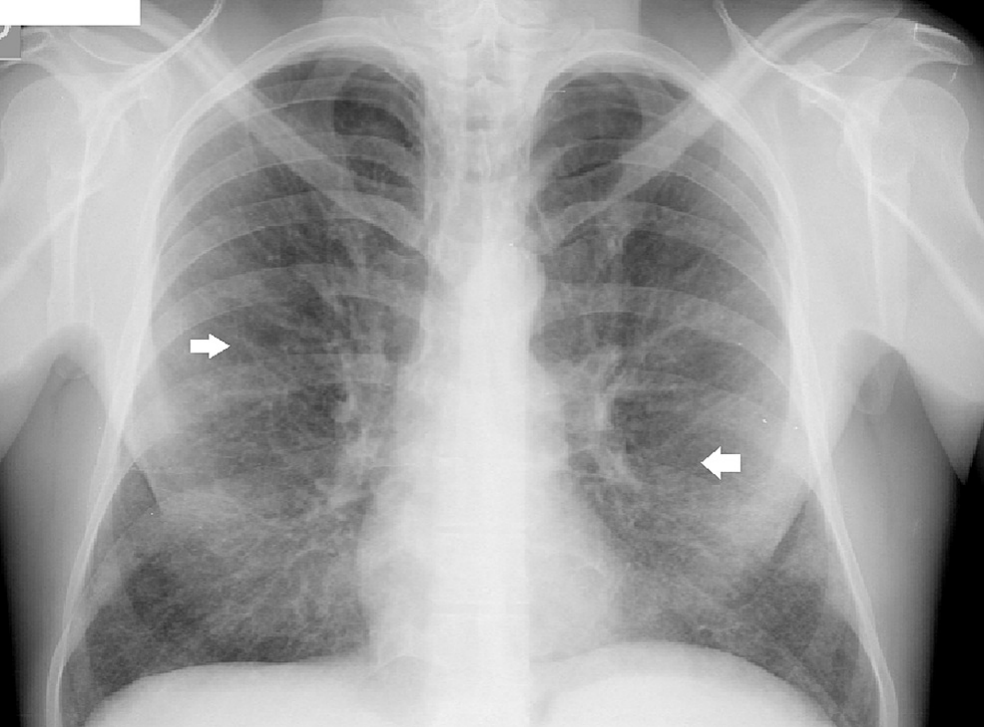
Chest radiography shows reticular infiltrates (white arrows).
